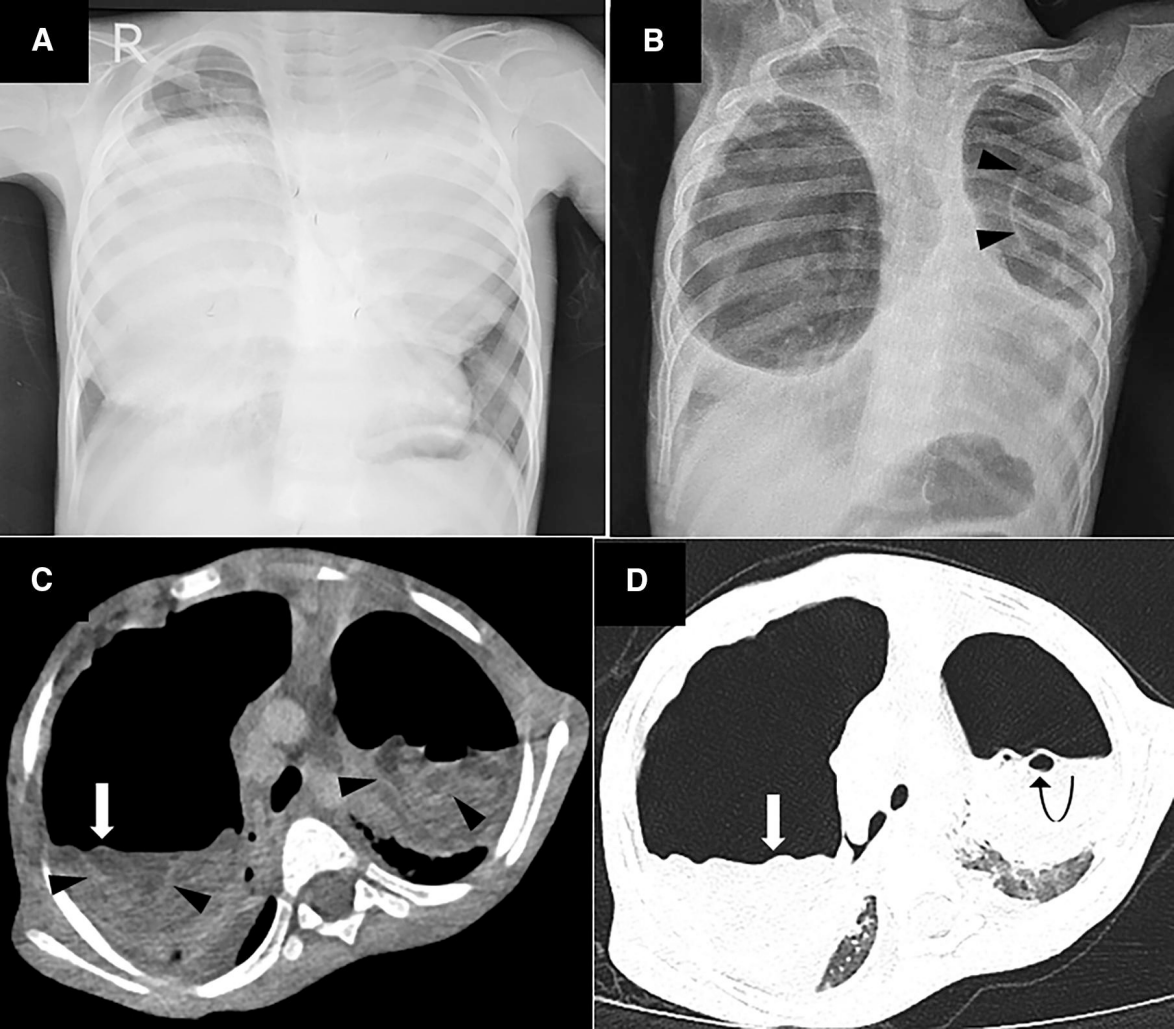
Chest radiographs and chest computed tomography (CT) scan of the child. (A) Initial chest radiograph showed large well-defined rounded opacities in bilateral lungs. (B) Repeat radiograph showed large, rounded air-containing cavities in bilateral lungs, with radio-opaque crumpled material within (Waterlily sign, arrowhead). (C) CT of thorax, mediastinal window, and (D) lung window showed well-defined bilateral cysts, with air-fluid level (arrow), with dependent wavy crumpled laminated contour, created by floating parasitic membranes (Waterlily/Camelotte sign; arrowhead) and air foci in the endocyst (air bubble sign; curved arrow).
